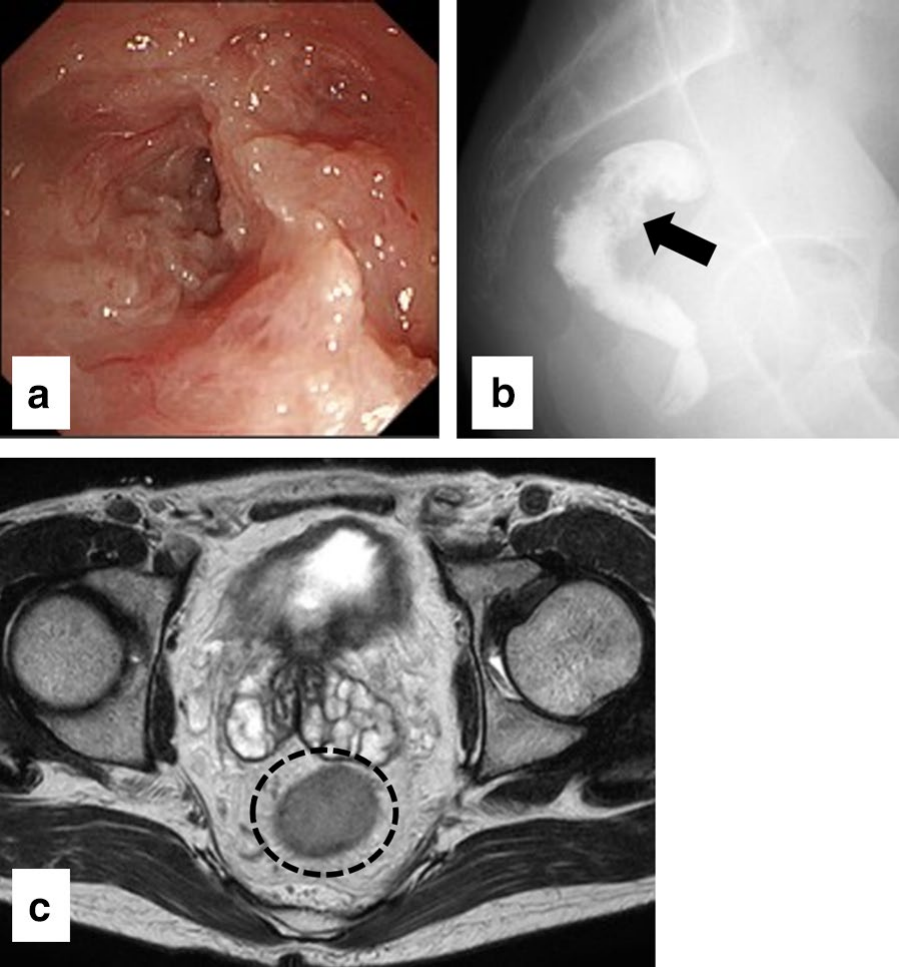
Preoperative examination findings. a Colonoscopy showed a circumferential tumor in the residual rectum. b Contrast enema showed the tumor located at the low rectum (black arrow). c Magnetic resonance imaging revealed the circumferential tumor at the low rectum (dashed circle). The depth of wall invasion by the tumor was estimated as T2 because the muscle layer of the rectum was continuous
